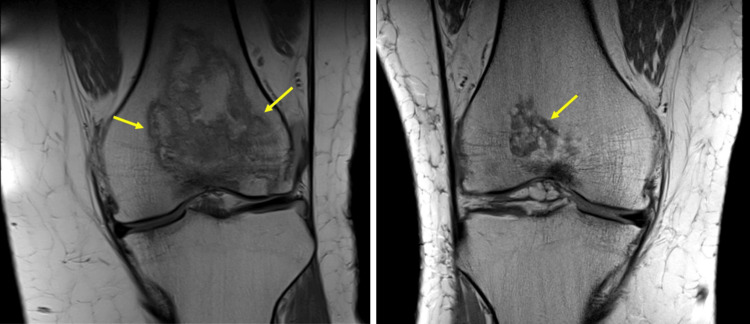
Bilateral knee osteonecrosis. Large bony infarcts are evident in both images. Note the serpentine low-signal intensity bands (arrowheads) surrounding the central area of necrosis on bilateral knee T1-weighted MRI.
